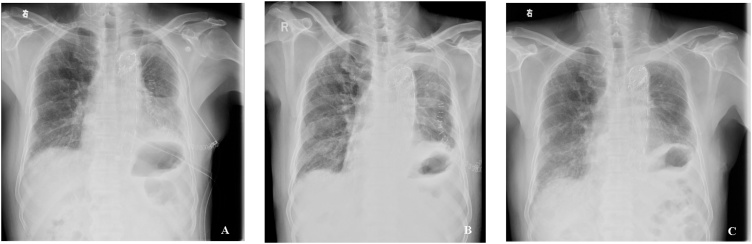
A. The chest X-ray on the day of discharge from the hospital (sixth postoperative day), at one month (2B) and four months after surgery (2C).
